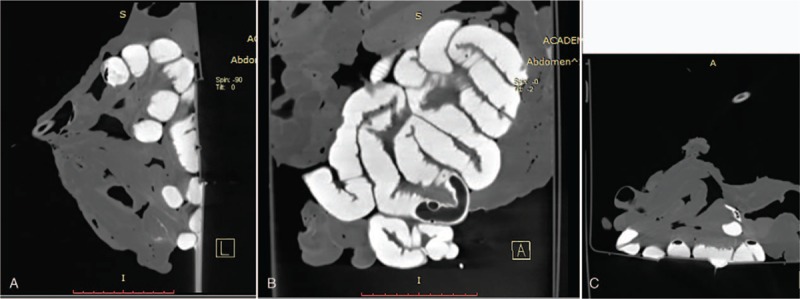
Coronal, sagittal, and cross-sectional screenshots of contrast-enhanced computed tomography images.
